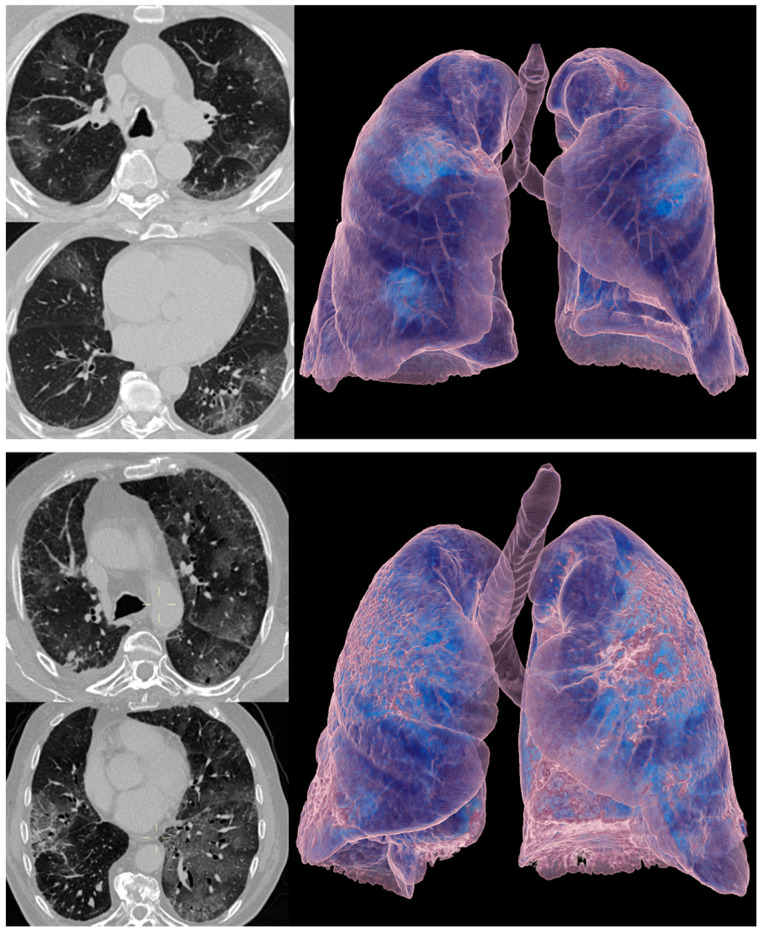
Computed tomography (CT) images of patients with RT-PCR-proven COVID-19 pneumonia (upper panel) and ICI therapy-related pneumonitis (lower panel). Axial CT images with lung window (left side of upper and lower panel) and 3D volume rendering technique (VRT) images (right side of upper and lower panel) are shown. Note the overlapping CT features of ground-glass opacities between the two cases of different etiology.
